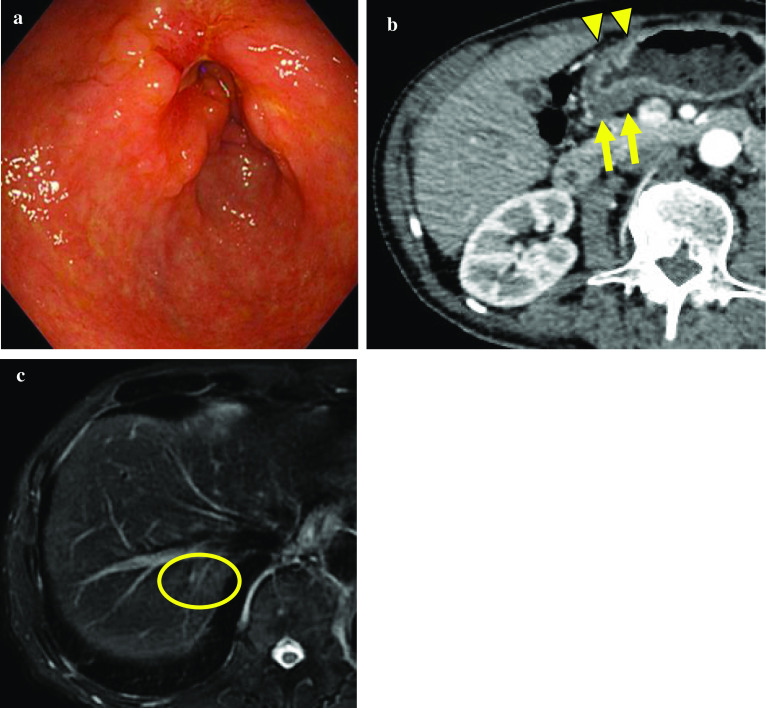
Image findings after preoperative chemotherapy. a Upper gastrointestinal endoscopy showed marked shrinkage of the primary tumor. b On CT, shrinkage of the primary tumor (triangle) and regional lymph nodes (arrow) was confirmed. c MRI showed marked shrinkage of liver metastasis (circle)
